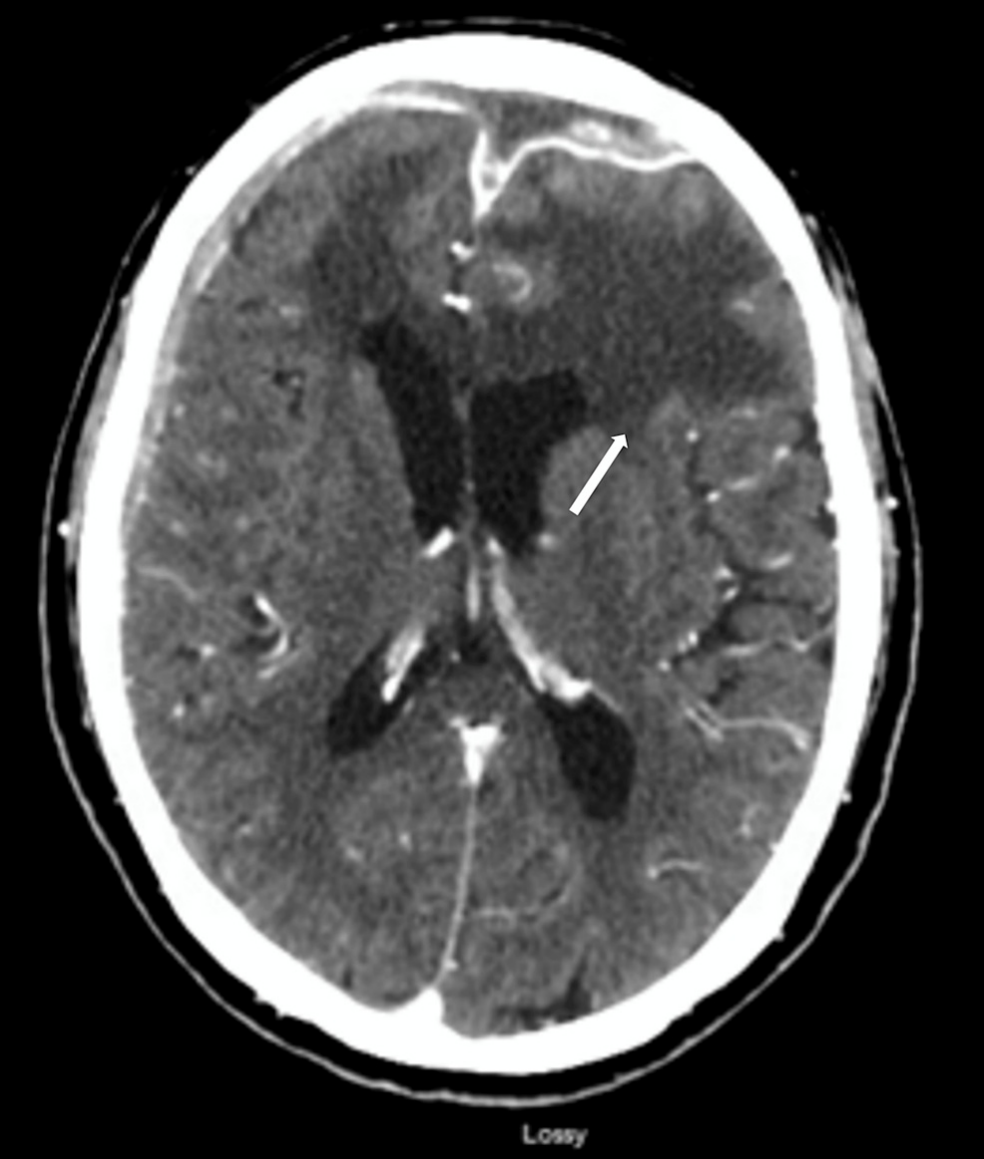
CT of the head displaying bifrontal subdural empyema
